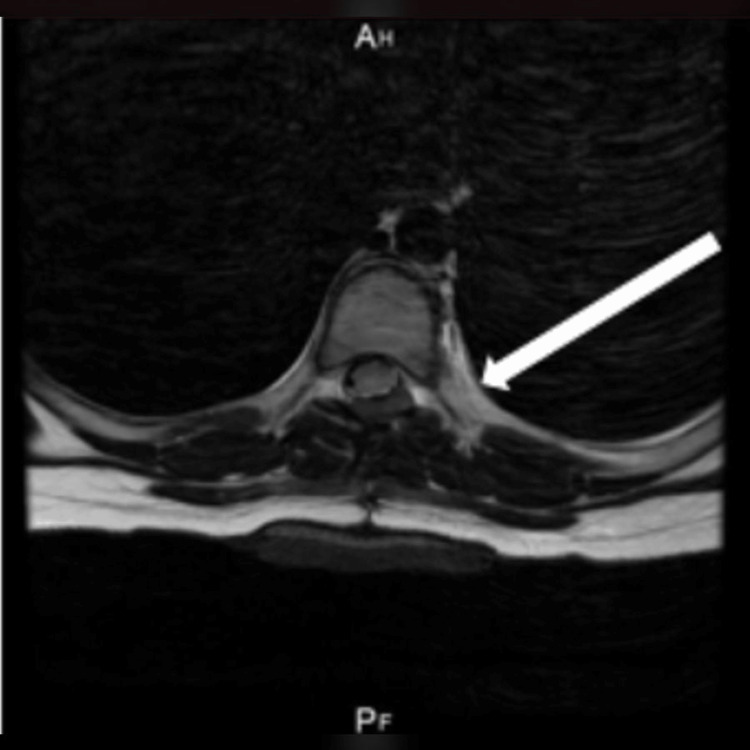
Spine MRI (T2 axial view) showing location where tumor was surgically removed (arrow).
